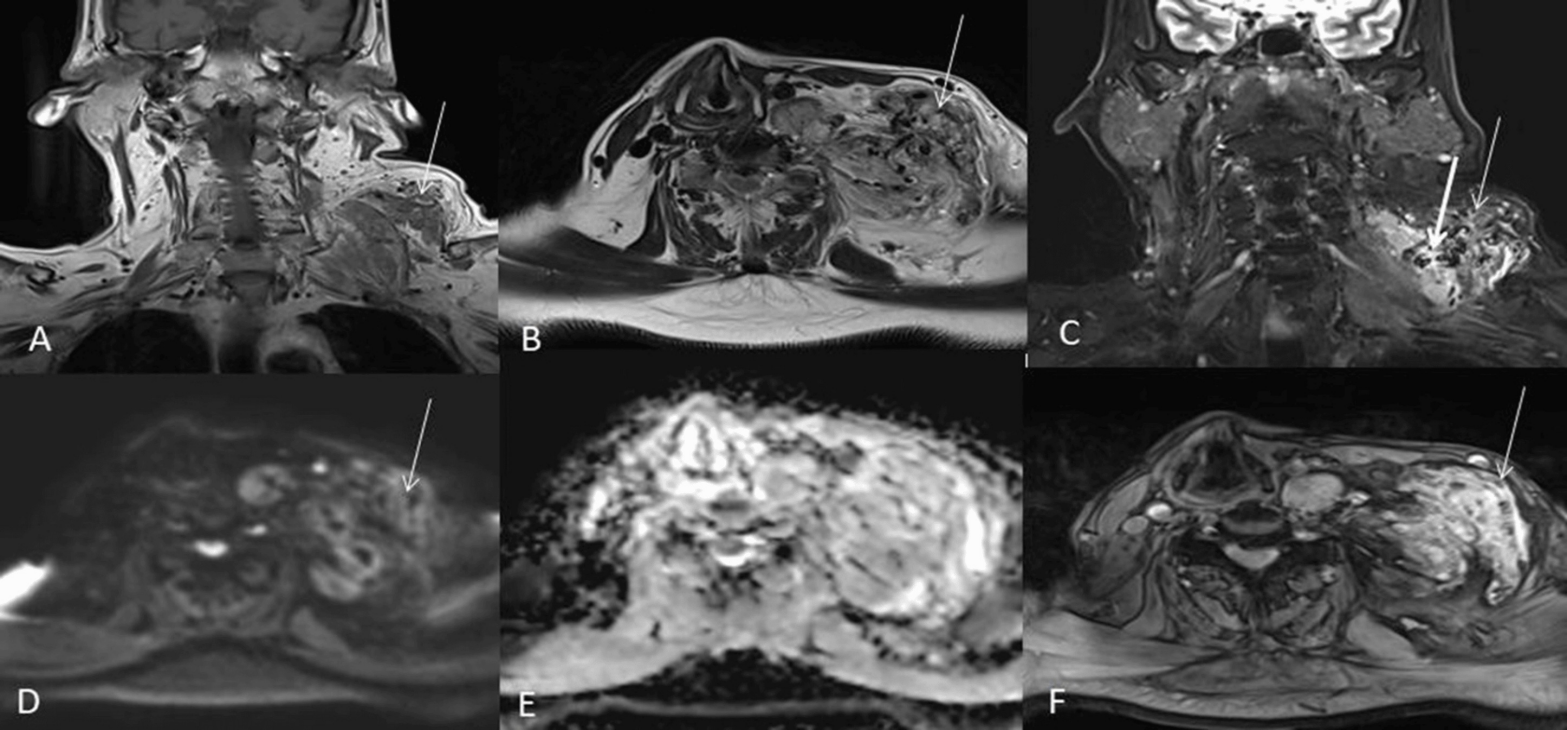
A male patient presented with left supraclavicular and neck swelling, predominantly in the deep subcutaneous and intermuscular plane. The swelling extended to the left paravertebral and posterior cervical space, causing a contour bulge in the left supraclavicular region. The lesion exhibited a predominantly isointense signal on T1-weighted imaging (T1WI) (A), with interspersed hyperintense areas likely representing fibro-fatty tissue. On T2-weighted imaging (T2WI) and short tau inversion recovery (STIR) sequences (B, C), the lesion appeared heterogeneously hyperintense. No diffusion restriction was observed (D, E). Multiple flow voids appearing hypointense were noted within the lesion on both T2WI and T1WI. Additionally, a few small low-signal intensity foci were seen within the lesion across all pulse sequences, showing blooming on gradient recalled echo (GRE), indicative of phleboliths (F).
